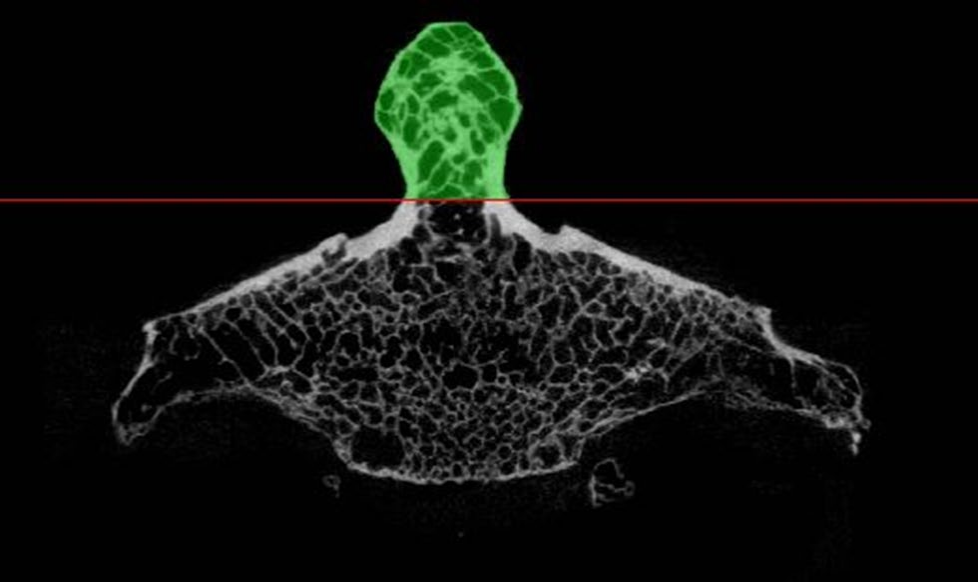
Region of interest for the assessment of the volumetric bone mineral density (vBMD) (green). The cross-sectional ratio of cortical bone to total bone (Ct.Ar/Tt.Ar) was assessed in the most caudal CT slice indicated by the red line
